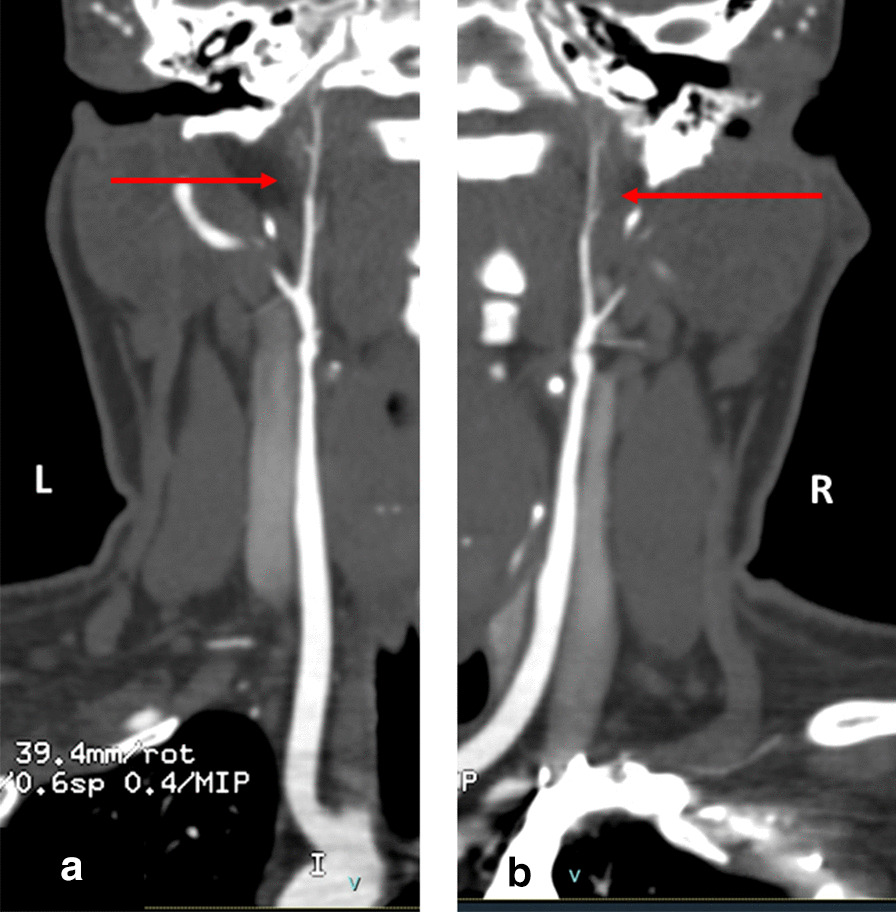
CT Angiogram of the neck with coronal reconstruction along course of the left (a) and right (b) carotid arteries of a 25year old female. There is marked stenosis of the bilateral internal carotid arteries (arrows) beginning distal to the carotid bifurcation
